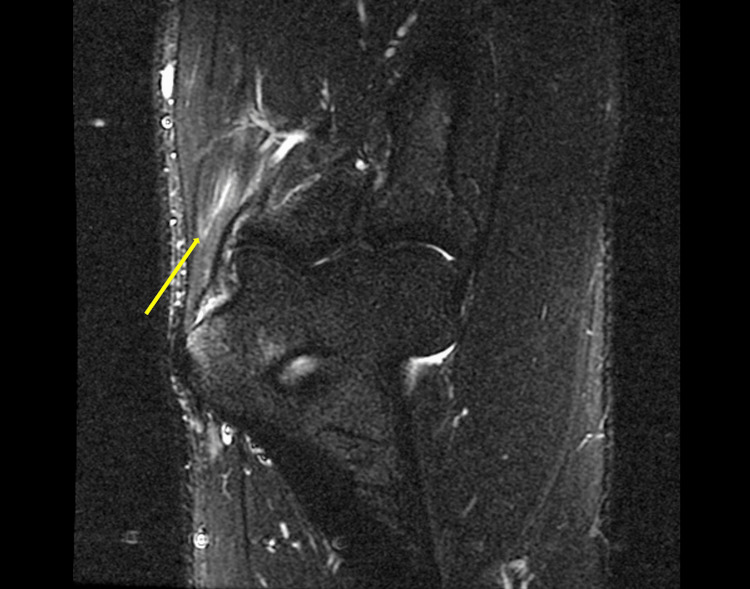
Coronal T2-weighted STIR MRI sequence showing hyperintense signal within the common flexor tendon fibres Credit: [18] STIR, short tau inversion recovery; MRI, magnetic resonance imaging
